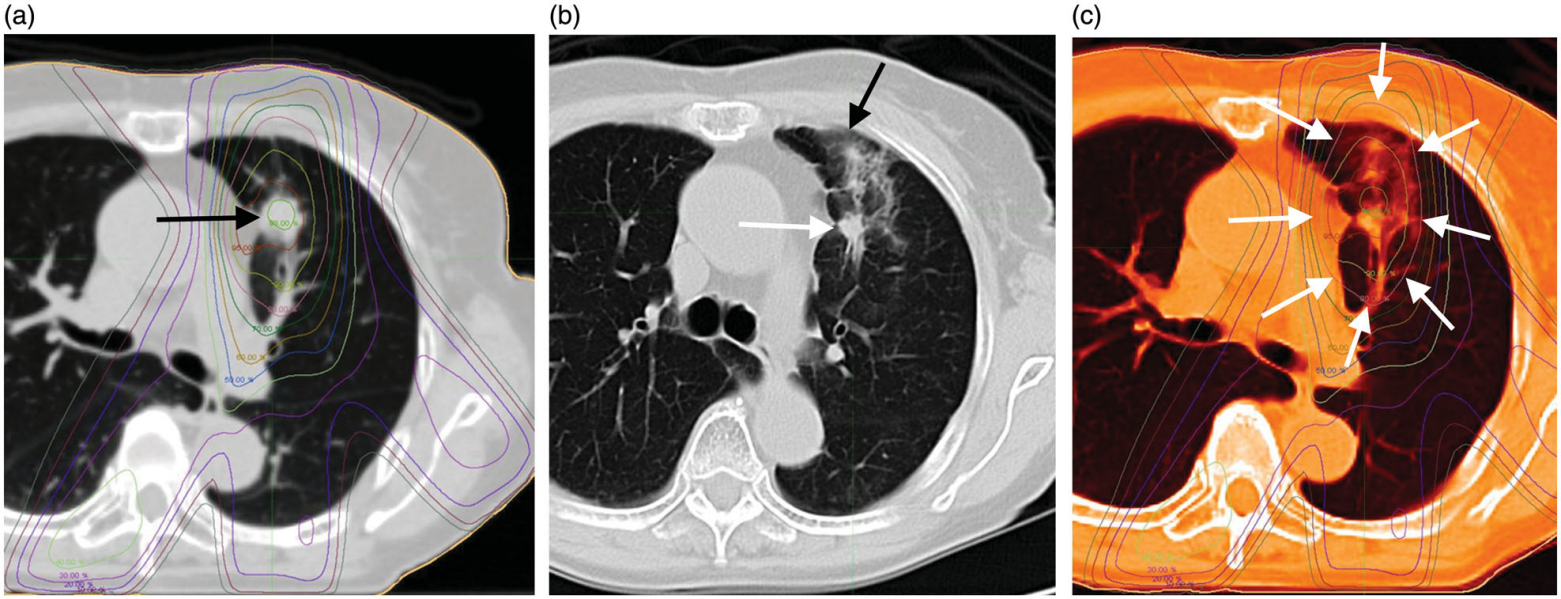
(a) The case is an 85-year-old woman with a 24-mm-diameter squamous cell carcinoma of the left upper lobe S3 lung (black arrow). (b) This shadow was diagnosed as a "Patchy consolidation and ground glass opacity (GGO)" in acute phase CT imaging at 114 days after radiotherapy (black arrow: ground glass opacity, white arrow: consolidation). (c) Treatment planning and acute computed tomography (CT) imaging were fused with MIM Maestro. The shadow of RILI was found in the 80% dose region (white arrows).
